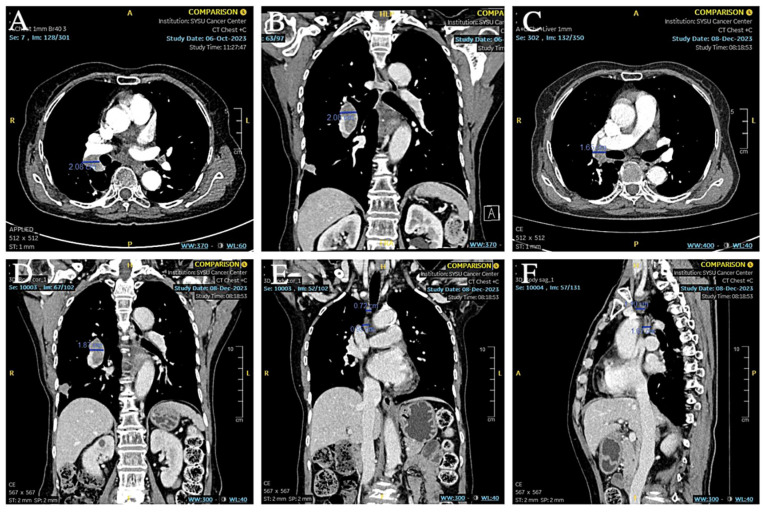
(A,B) CT showed that the hilar lymph nodes were smaller than before. (C–F) CT showed that the short diameter of hilar lymph nodes was reduced to about 19 mm, and other mediastinal lymph nodes were also reduced from the previous size.
